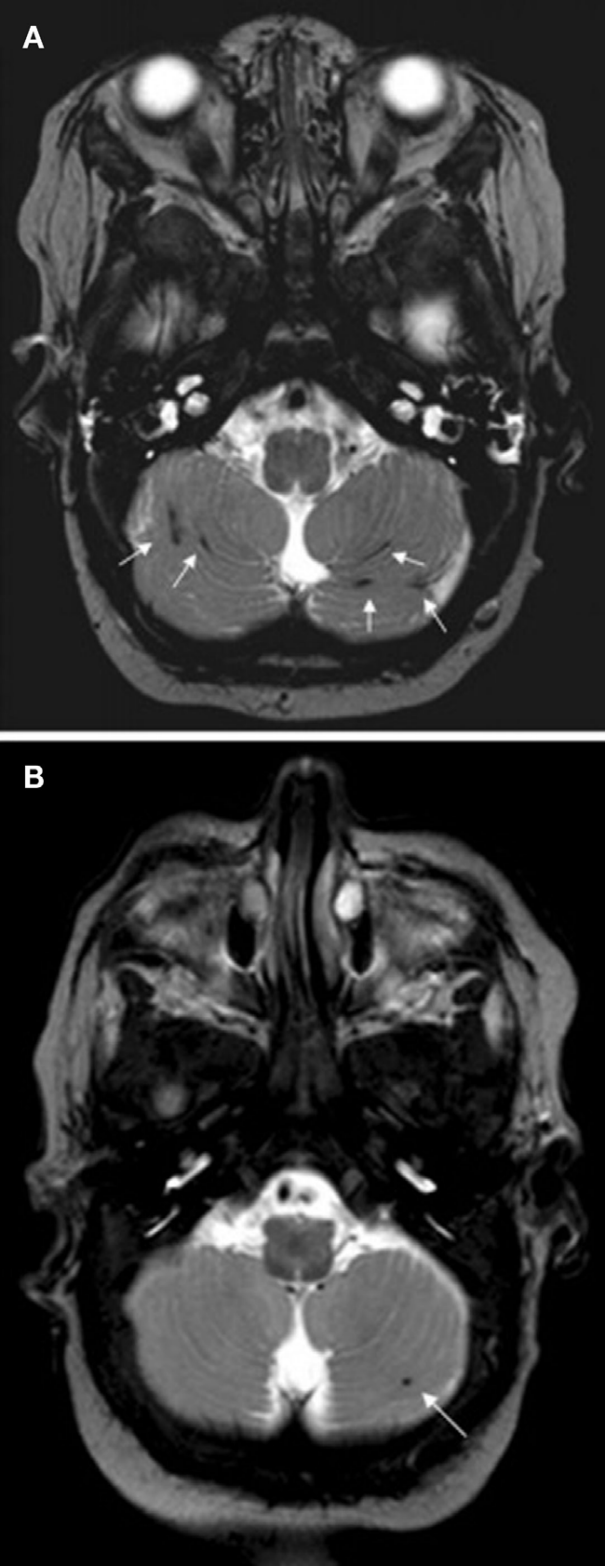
Two preterm infants born at 29 weeks gestational age, imaged at term with T2 weighted MRI. Subject A exhibited multiple small streak-like hemorrhages in both cerebellar hemispheres (see arrows). Subject B exhibited a single small hemorrhage in the left cerebellar hemisphere (see arrow). Figure was reproduced with permission (61).
